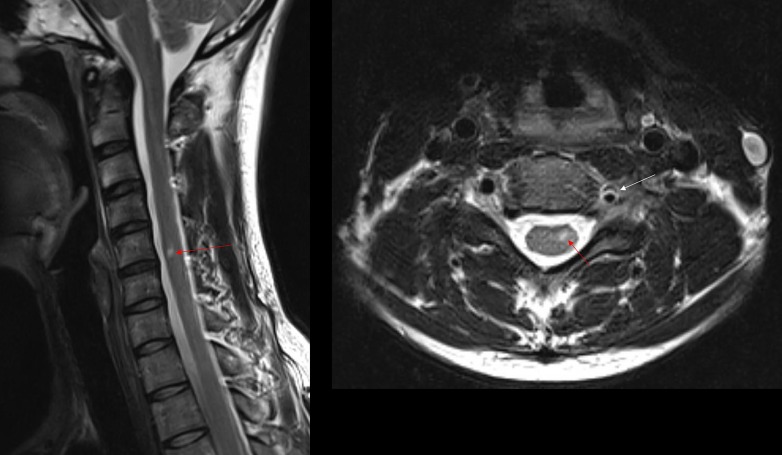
Sagittal and axial T2-weighted images demonstrate hyperintensity within the left hemicord at the C5 level (red arrow) Note that the left vertebral artery flow void is irregular and narrowed (white arrow).
